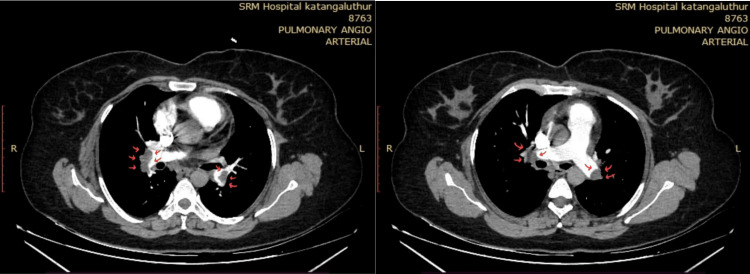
CT pulmonary angiogram showing partially occluding hypodense filling defects in right and left pulmonary artery and in segmental divisions (red arrows).
